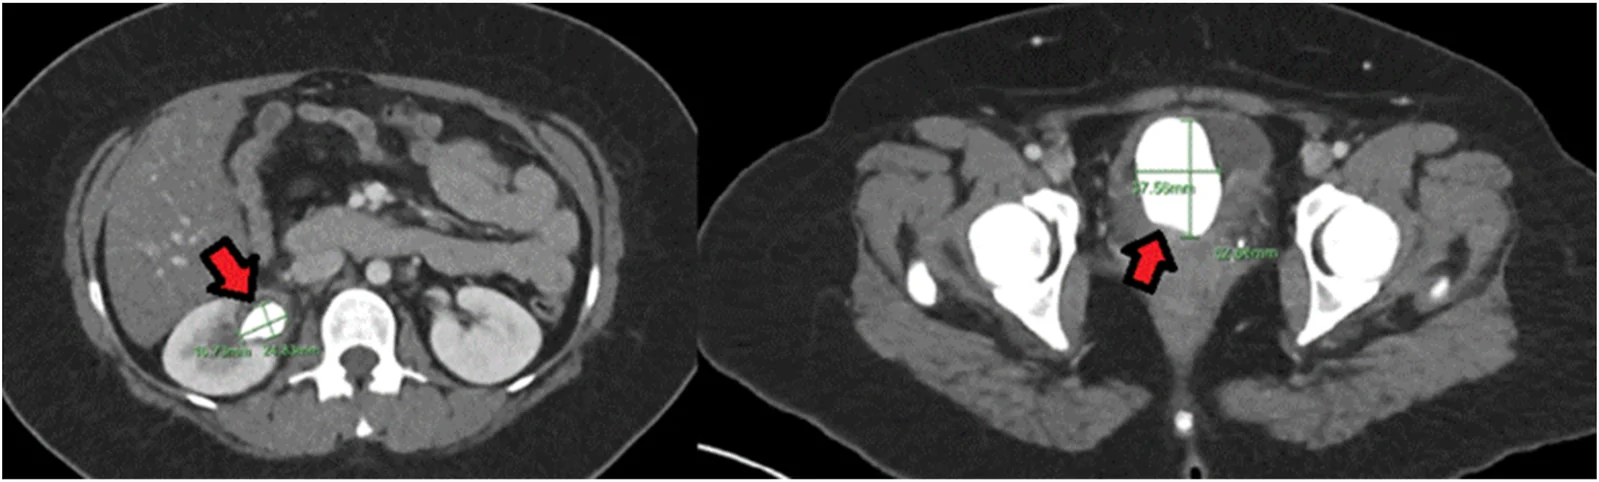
Axial contrast-enhanced CT of the abdomen and pelvis demonstrating a right-sided renal and bladder calculi with a retained ureteral stent.
